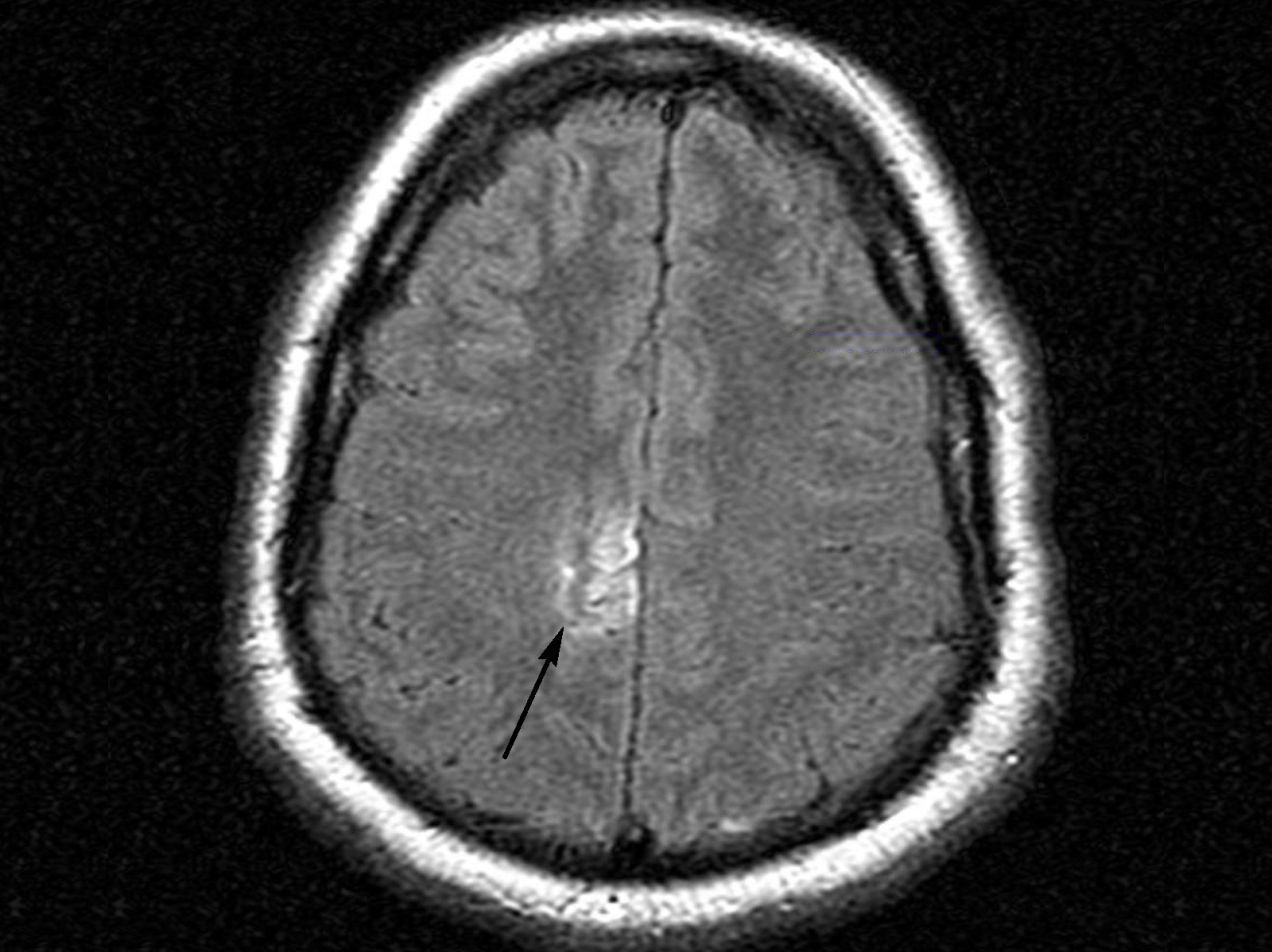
MR FLAIR demonstrating cortical laminar necrosis
